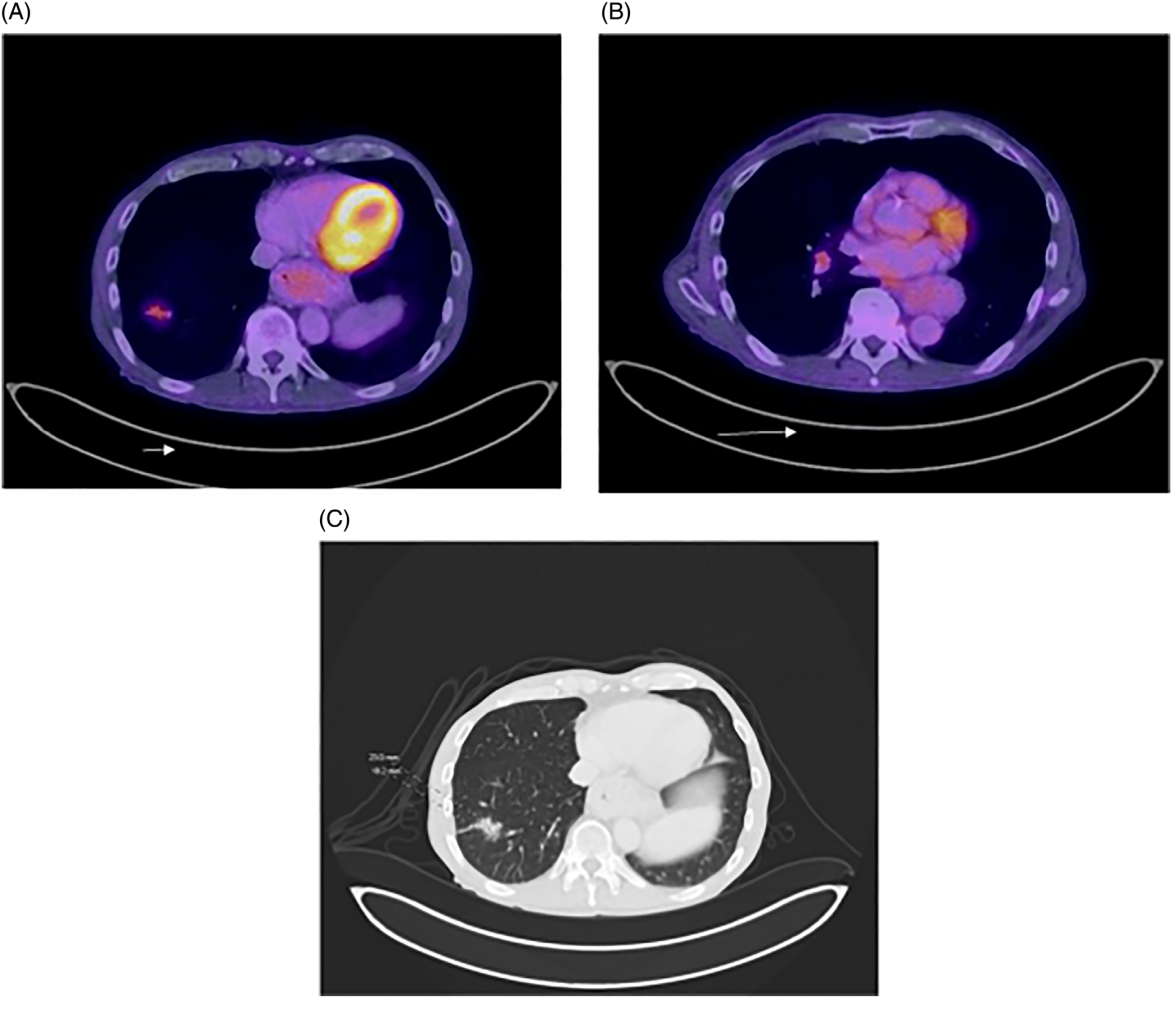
PET/CT showing right lung nodule A, and right hilar lymph node B. CT imaging of the spiculated lung nodule C
